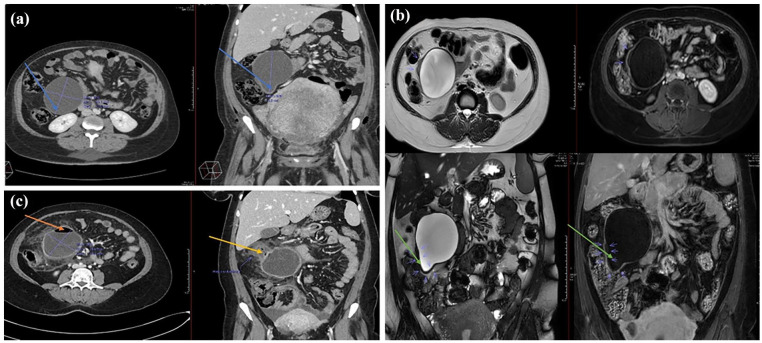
(a) CT scan showing the transverse section (left) and frontal view (right) of the abdomen. The cyst, with a caudal protrusion and focal wall thickening, was present on the right side of the abdomen (blue arrows). (b) MRI scan with transverse section (above) and frontal view (below) of the abdomen showing the cyst with a smooth wall and caudal protrusion with focal wall thickening (green arrows). The cyst contents were mostly homogenous with high T2 and low T1 signal intensities and were diagnosed as a benign cyst, possibly lymphangioma. (c) CT scan of the abdomen showing air content in the cyst (orange arrow on left image) and area of rupture and reactive inflammation (yellow arrow on right image).
